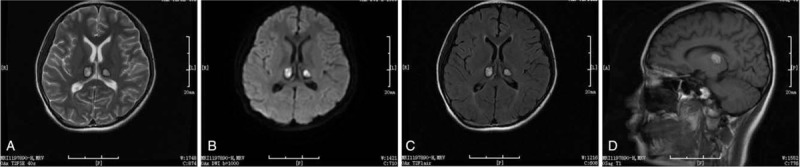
Magnetic resonance imaging of the same patient 15 days after diagnosis with acute necrotizing encephalopathy. The scans showed reduction in the lesions of bilateral dorsal area of the thalami, dorsal caps of the pontine, and bilateral cerebellar hemispheres. Short T2 signals around the lesions are also evident. (A) T2. (B) Diffusion-weighted imaging. (C) T2 flair. (D) T1.
